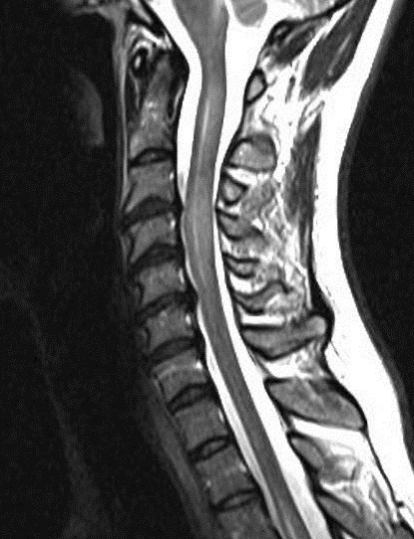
MRI spine showing hyperintensity in cervical cord C5 to C7 level (1)
